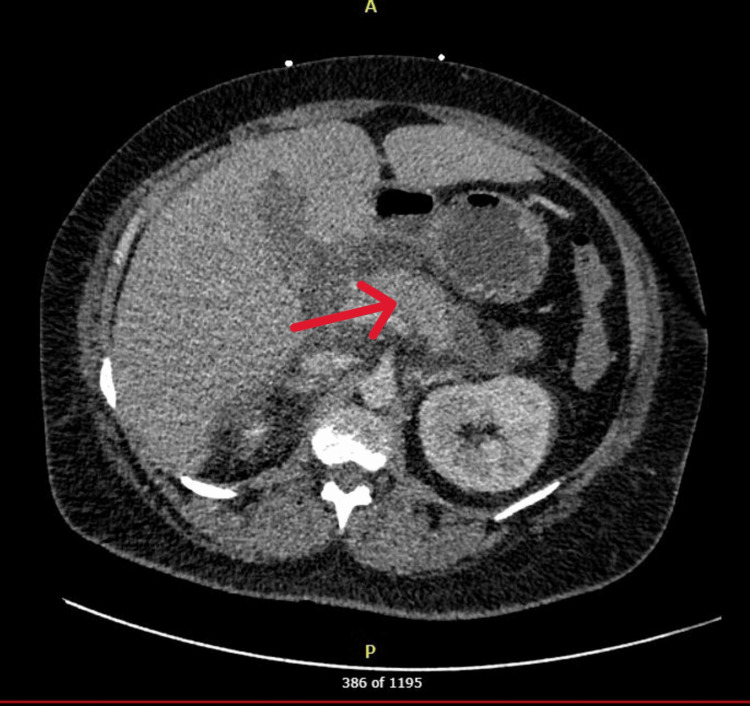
CT scan of acute pancreatitis from case report Radiology report of CT scan. Edematous appearance of the pancreas with severe surrounding inflammatory changes with fluid tracking in the mid abdomen, right paracolic gutter, and right greater than left anterior pararenal spaces. Mild inflammatory changes in the right perirenal space. Findings consistent with acute interstitial edematous pancreatitis indicated by the red arrow.
